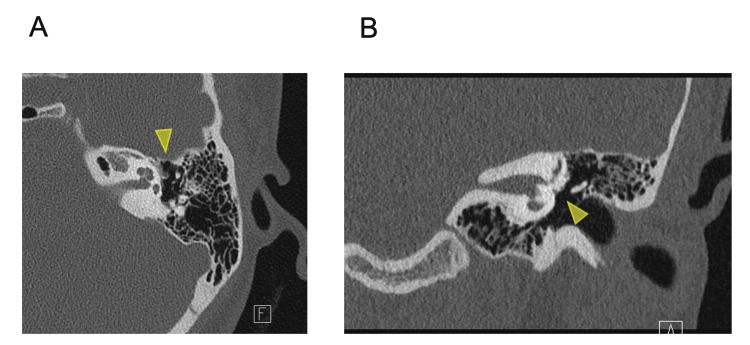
CT scan findings of the patient. CT-scan (A: horizontal section, B: coronal section) showing suspected middle ear anomalies with potential incus-stapes joint deficiency (triangle).
